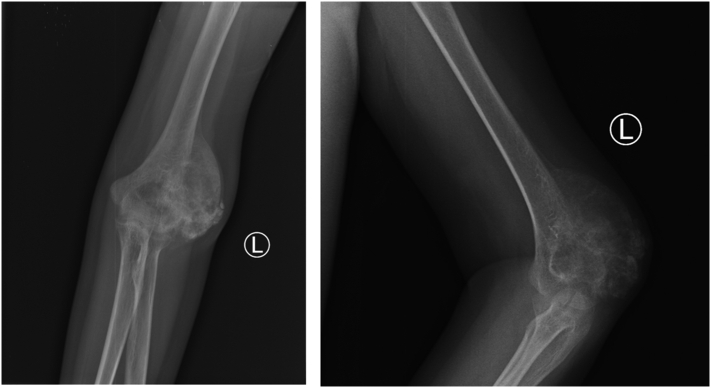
X-ray examination of left elbow showing osteolytic lesion and periosteal reaction.
